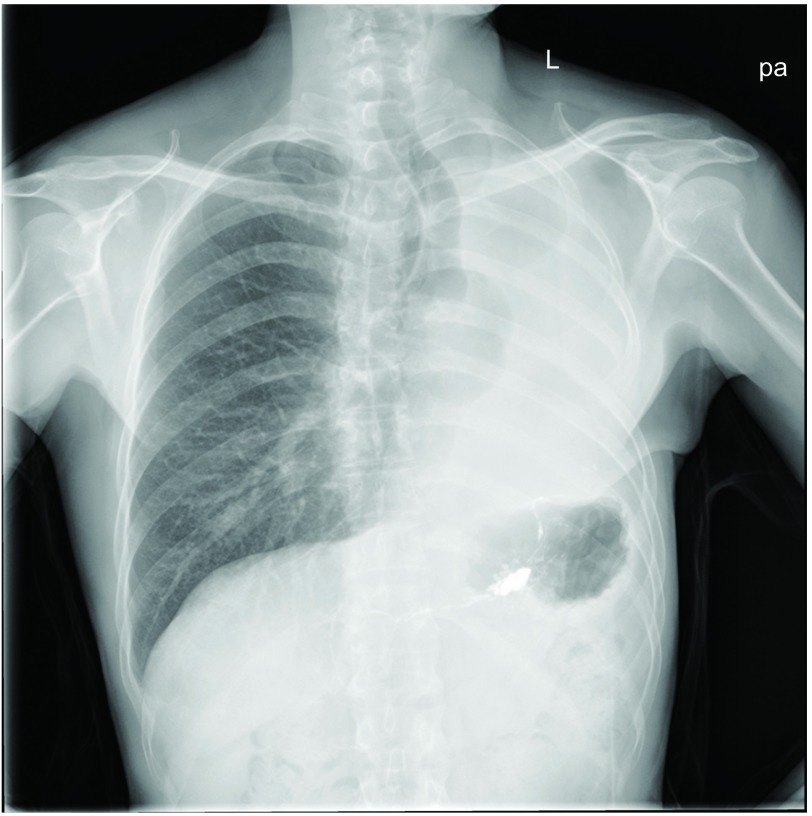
患者术后胸片（2015年5月），显示左全肺切除术后的状态，气管左偏，右肺野很清晰。 Chest X-ray after operation eight months (May in 2015), which shows a state of left pneumonectomy, trachea shift to the left, and right lung field is very clear.
